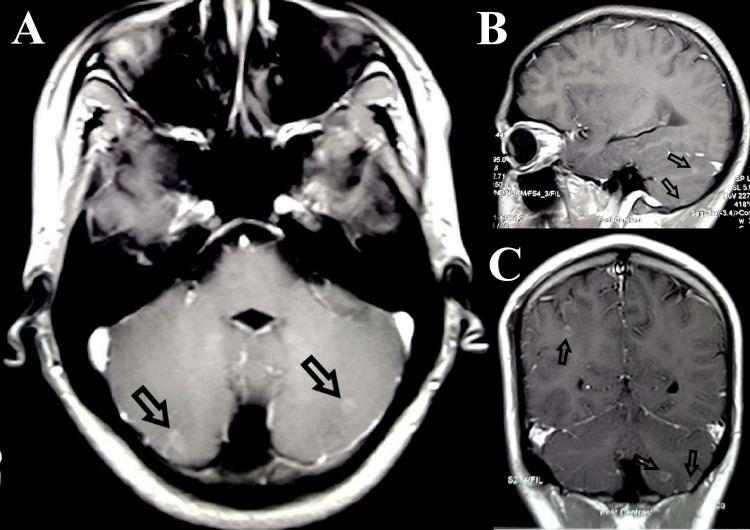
Transaxial (A), sagittal (B), and coronal (C) post Gadolinium T1 weighted MR image of the brain showing multiple supra and infratentorial peripherally enhancing tuberculomas (arrows)
